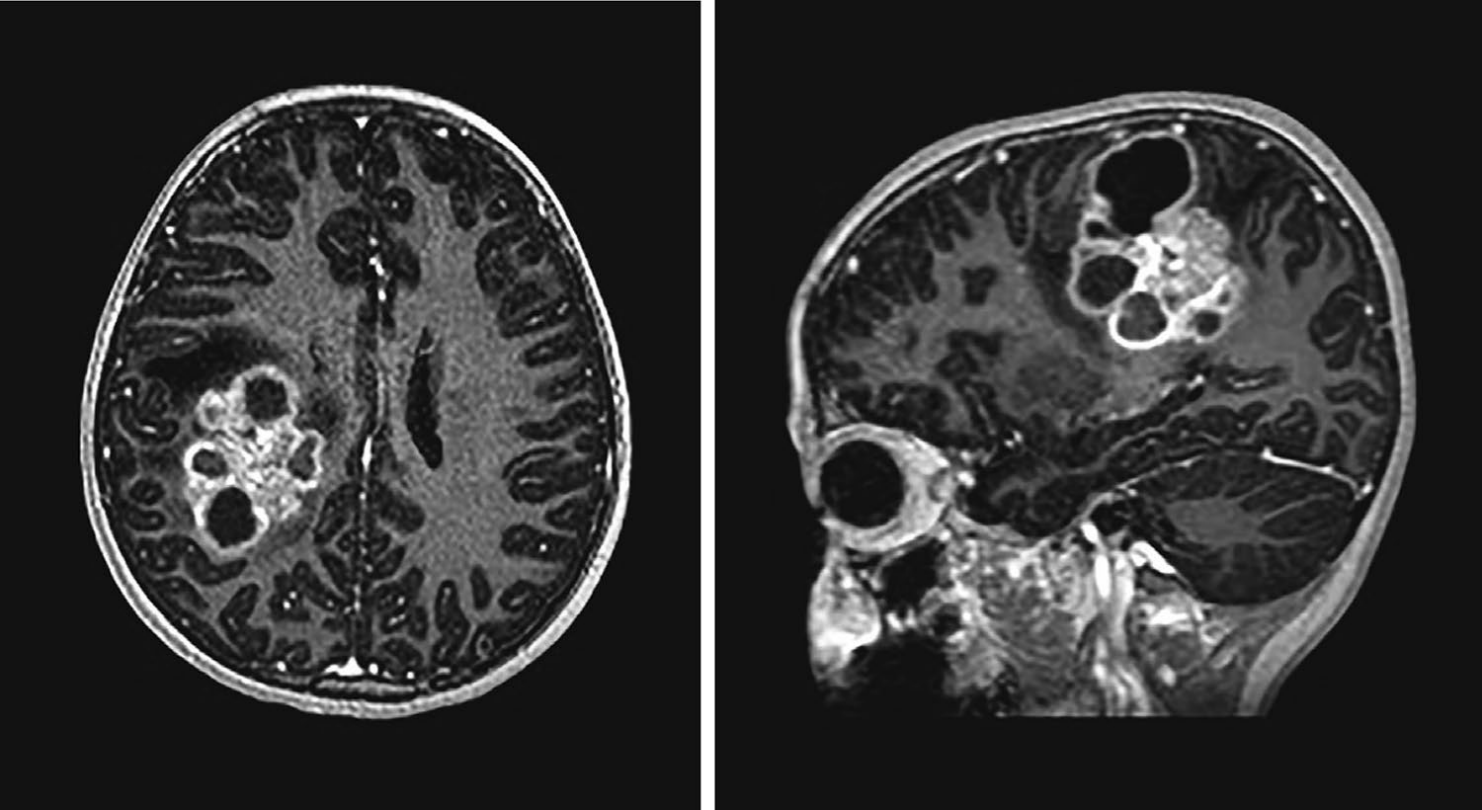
Typical MR findings of EET MN1-BEND2. T1 post-contrast MR images of EET MN1-BEND2 presenting in a 9-year-old girl. T1 post-contrast axial and sagital images show typical well-demarcated complex solid and cystic appearance of EET MN1-BEND2. Like other supratentorial ependymal tumors, they often show a bubbly and/or multinodular appearance [59]. Images courtesy of Dr. Bret Mobley, Vanderbilt University
